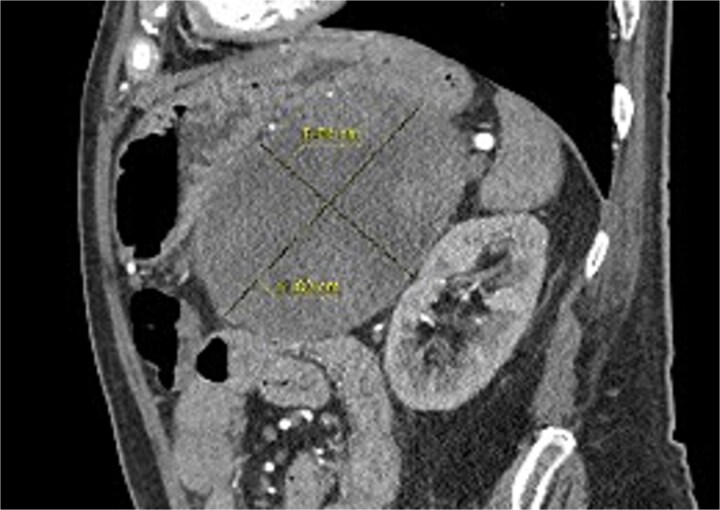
Sagittal high resolution CT of the PACC prior to surgery.
